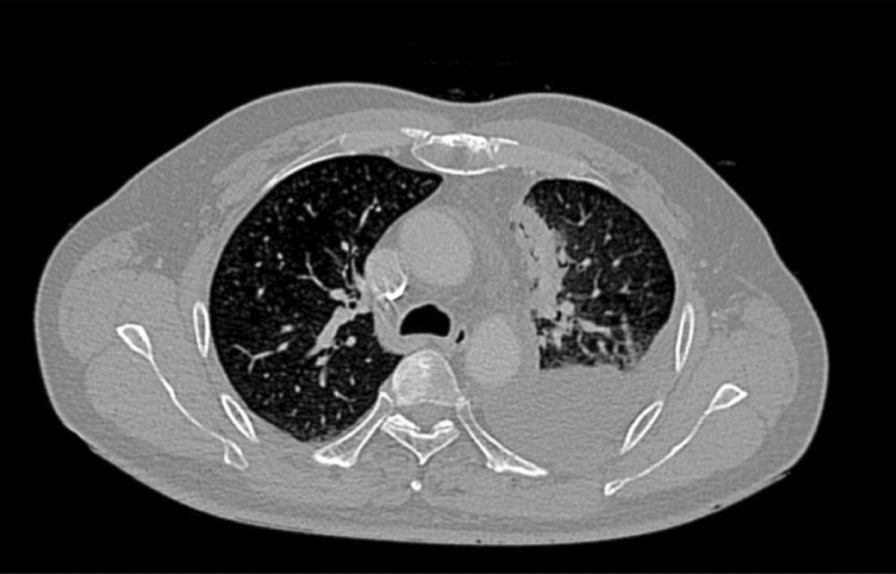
The chest CT scan showed pleural effusion and mass expansion. Accumulation of pleural effusion and expansion of solid mass in the left thorax by 39 months
